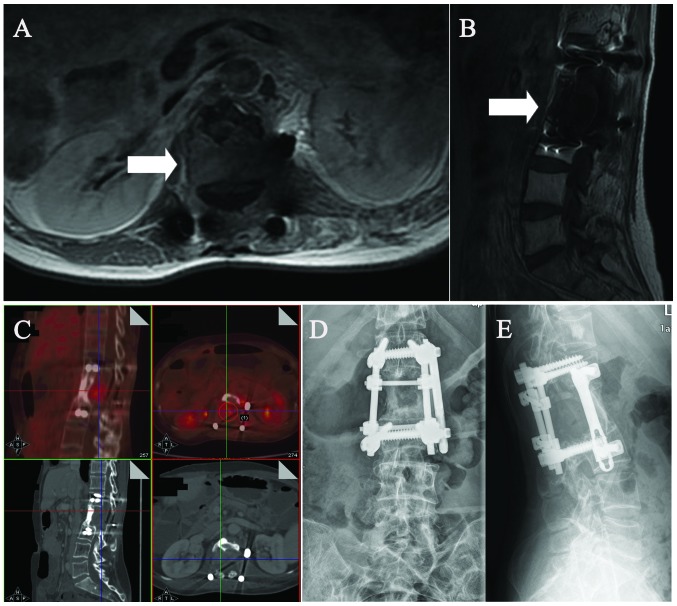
Pre- and post-operative radiographic images of the L2 metastatic malignant solitary fibrous tumour. (A and B) Magnetic resonance imaging scan revealing a posterior enhancing mass and spinal cord compression at the L2 level (arrows). (C) Positron emission tomography-computed tomography scan revealing a focal high uptake in the L2 level. (D and E) Post-operative radiograph subsequent to posterior palliative resection of the lumbar mass and re-fixation of larger pedicle screws. L2, lumbar 2.
